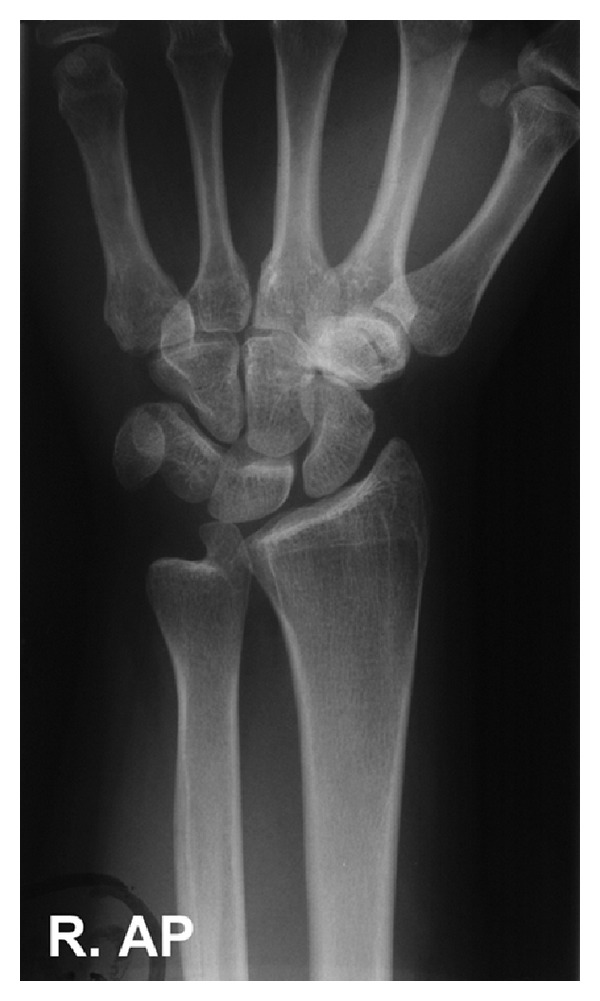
On arrival AP radiograph of the injured right wrist demonstrating the abnormal appearance of the ulnar styloid: it is lying adjacent to the ulnar notch of the radius.
